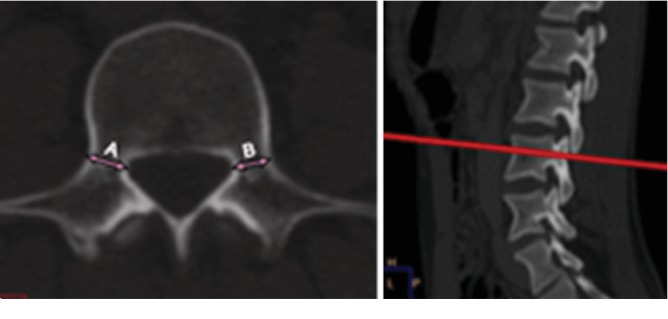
Measurement of pedicle width as conducted on an axial CT scan (a) at the middle height of the pedicle (b).
